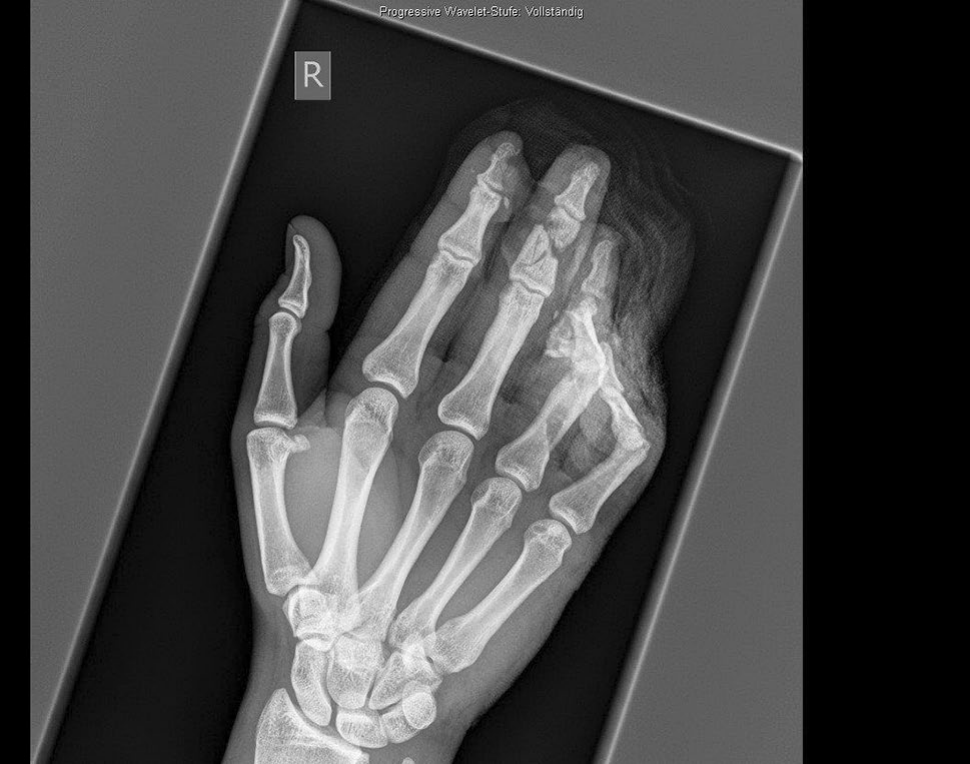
Case Presentation 2: X-ray after circular saw injury
